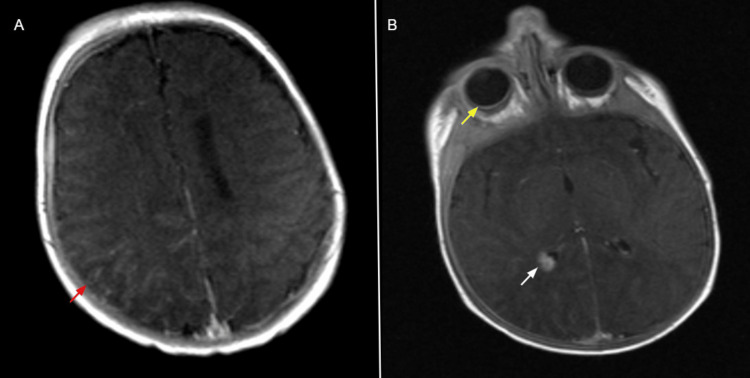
Contrast-enhanced MRI brain image at one month of age Post-contrast T1-weighted non-fat-saturated axial image (red arrow in A) demonstrates enhancing pial angiomas over the right cerebral hemisphere. Post-contrast T1-weighted non-fat-saturated axial image (yellow arrow in B) shows a diffuse enhancing choroidal hemangioma of the right eye and  (White arrow in B) indicates asymmetric enlargement of the right choroid plexus, confirming the diagnosis of Sturge–Weber syndrome.
